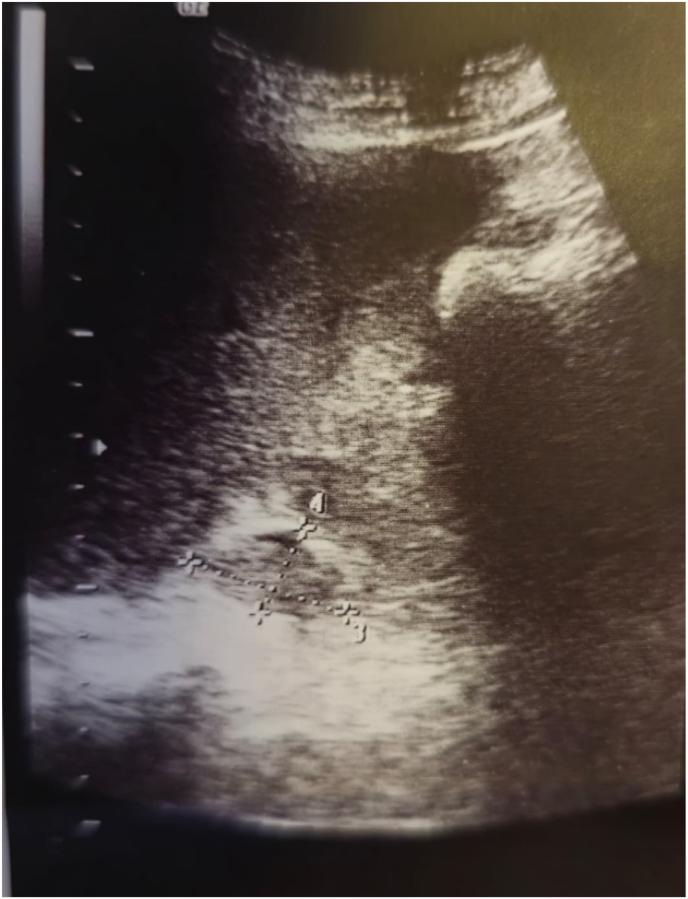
The ultrasound revealed a well-defined, solid hypoechoic mass in the right adrenal gland, measuring approximately 32 × 19 mm.
